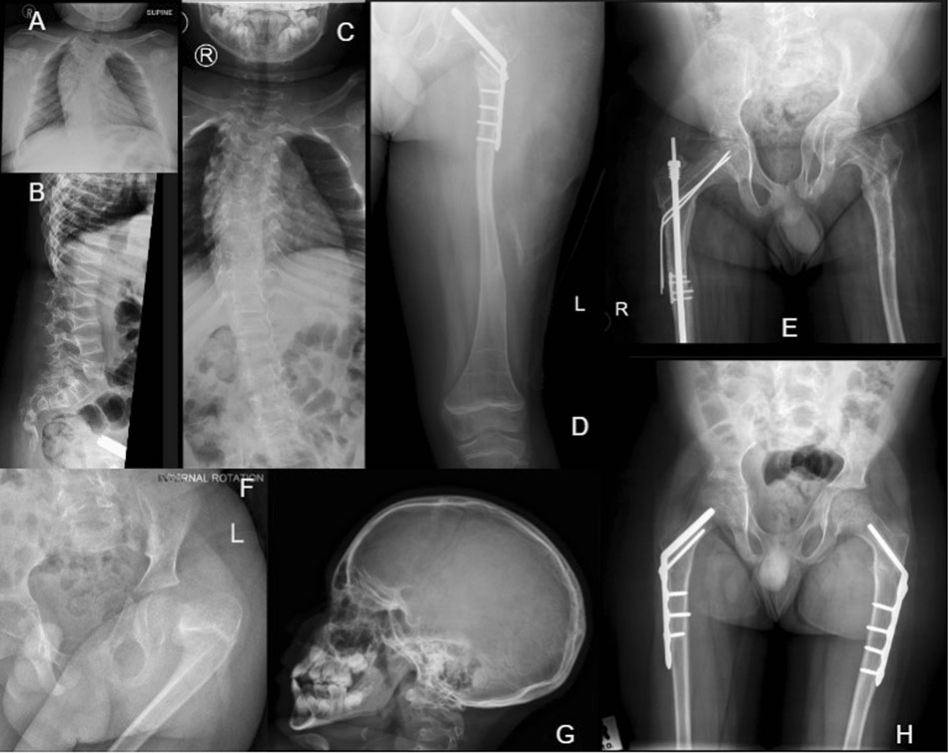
Radiographs of patient 14 (*FKBP10*). A AP chest showing scoliosis at age 9 years. B Lateral spine showing wedge fractures of the lumbar vertebrae at age 9 years. C AP chest showing scoliosis and abnormal vertebrae at age 10 years. D Left leg showing mild deformity, thin femoral shafts, zebra stripes from bisphosphonate therapy, and orthopaedic nailing of femoral head at age 12 years. E AP pelvis and proximal femora showing asymmetrical pelvis, thin femoral shafts, osteopenia and rodding of the right femur at age 13 years. F Fracture and deformity of left femoral head at age 5 years. G Lateral skull showing numerous Wormian bones at age 9 years. H AP pelvis and proximal femora with corrective orthopaedic nails and plates at age 7 years.
